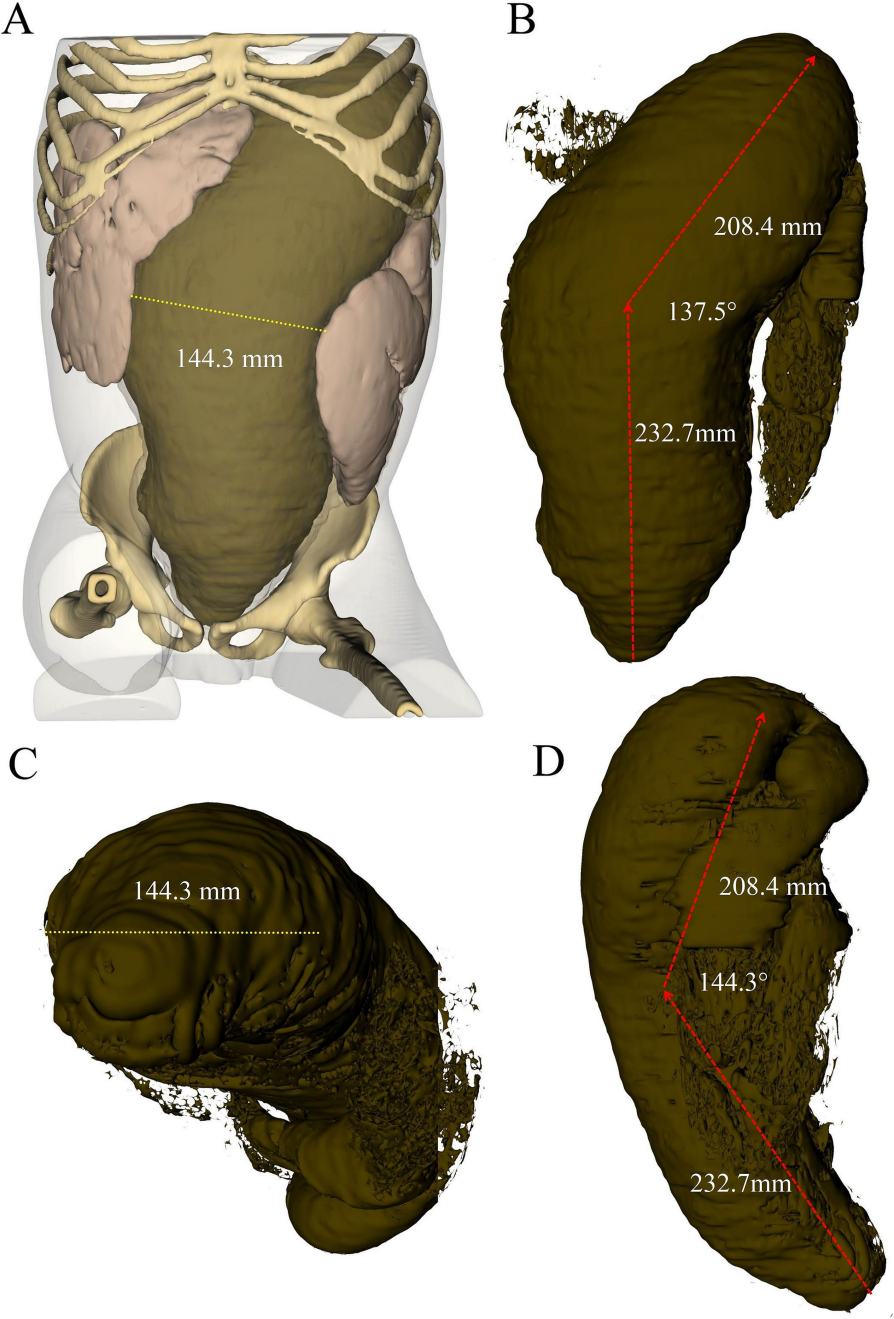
Three-dimensional reconstruction of abdominal CT scan depicting fecalith morphology from different perspectives: (A) overall three-dimensional model of the abdomen, (B) coronal view, (C) axial view, and (D) sagittal view.
